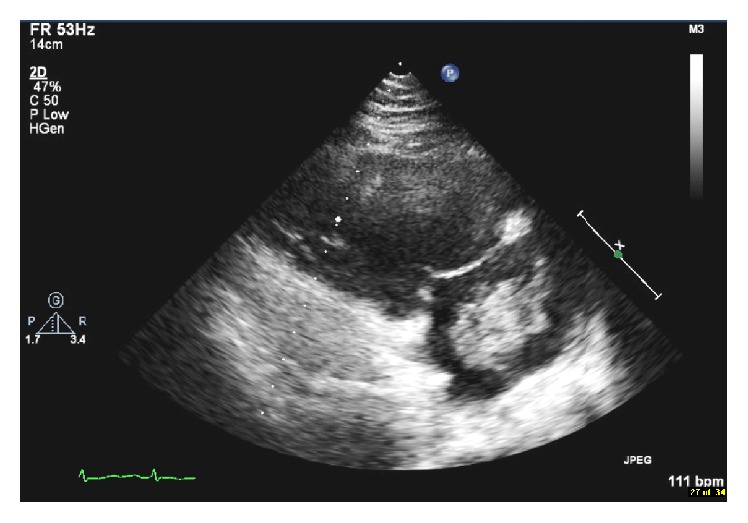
Echocardiogram showing large thrombus in the right atrium.
